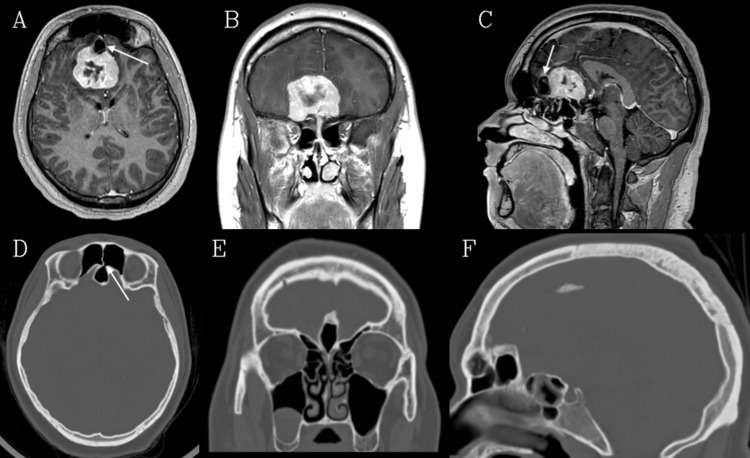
Preoperative imaging A, B, C: Axial, coronal, and sagittal T1 contrasted images, respectively, showing the extent of the OGM. The aerated CG is also visible on the axial and sagittal images, sitting against the anterior border of the tumor (arrows). D, E, F: Axial, coronal, and sagittal CT images (bone window), respectively, demonstrating the characteristics of the CG. Panel D shows how it derives its aeration from the left frontal sinus (arrow). It can be classified as type I, given its growth entirely above the cribriform plate.
